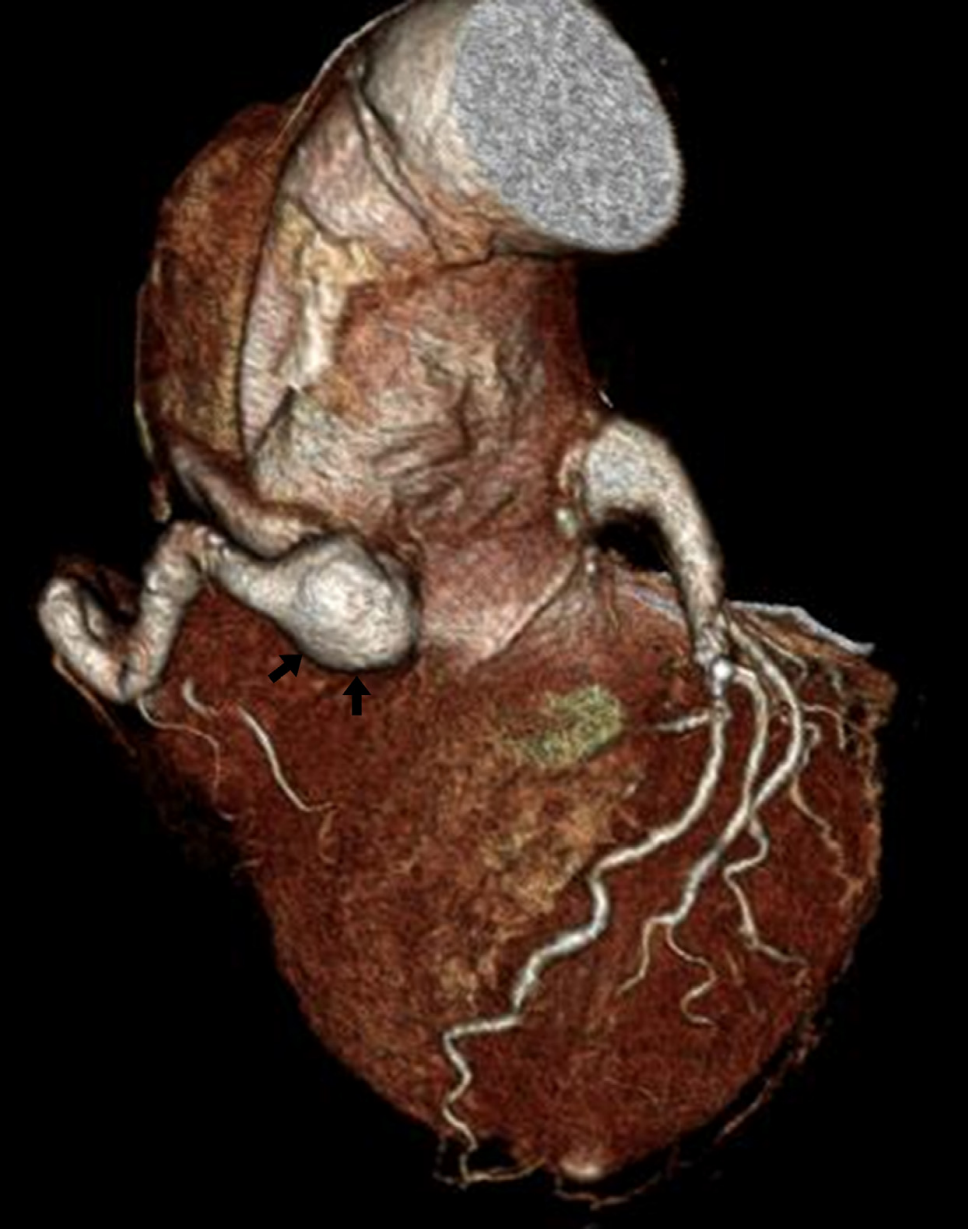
Chest CT before the third operation. The anastomotic site of the right coronary artery after the Cabrol technique is markedly enlarged (black arrows), while that of the left coronary artery is slightly enlarged.
